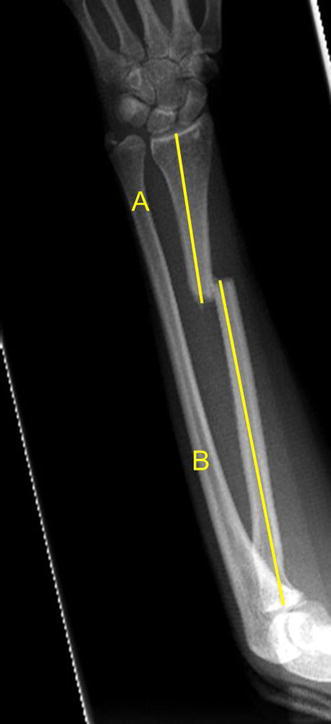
PA forearm radiograph: a ratio using the distance from the RCJ to the fracture divided by the entire length of radial shaft was then calculated to account for variations in the size of patient’s forearms (*a*/*a* + *b*)
